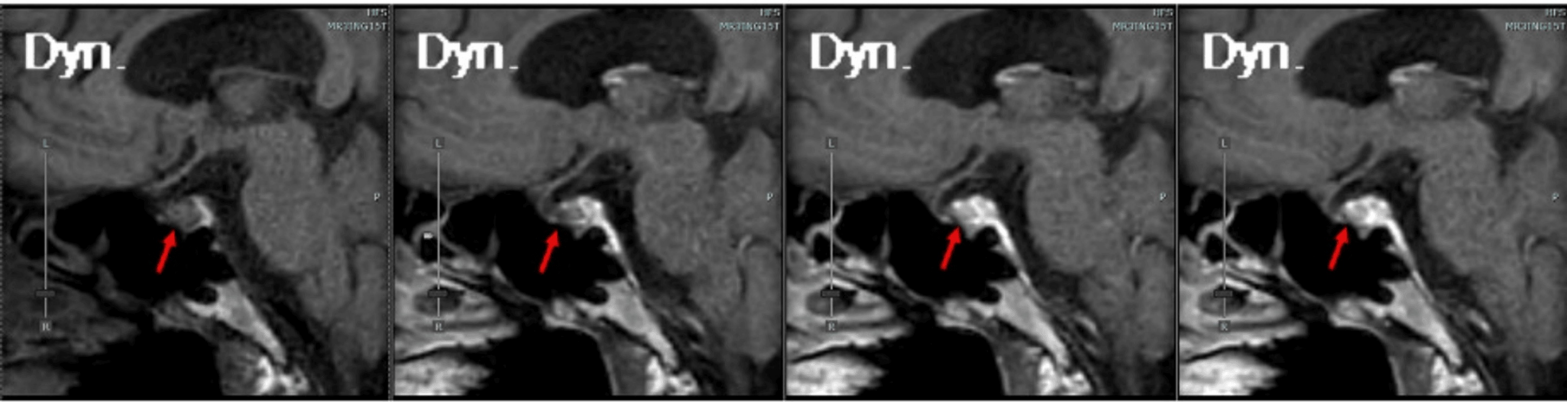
Sagittal view of contrast-enhanced pituitary MRI. Sagittal view of contrast-enhanced pituitary MRI, progressing from early phase to late phase, from left to right. The red arrow points to the pituitary gland, and there are no obvious abnormalities.
